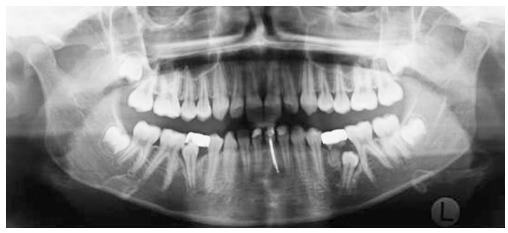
Pretreatment (T_1_) panoramic radiograph.
